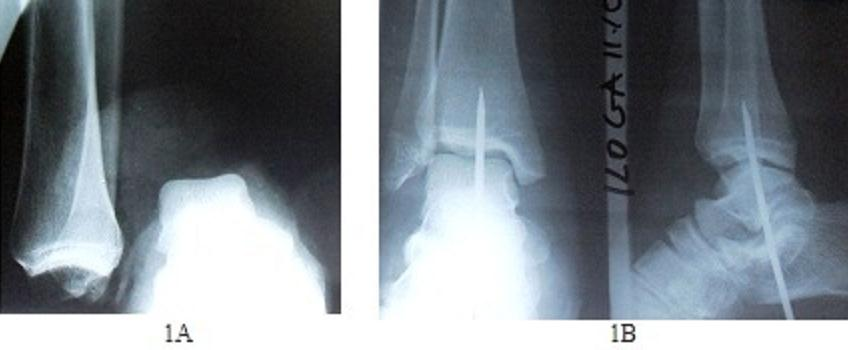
Radiographies (1A Préopératoire; 1B Postopératoire)
